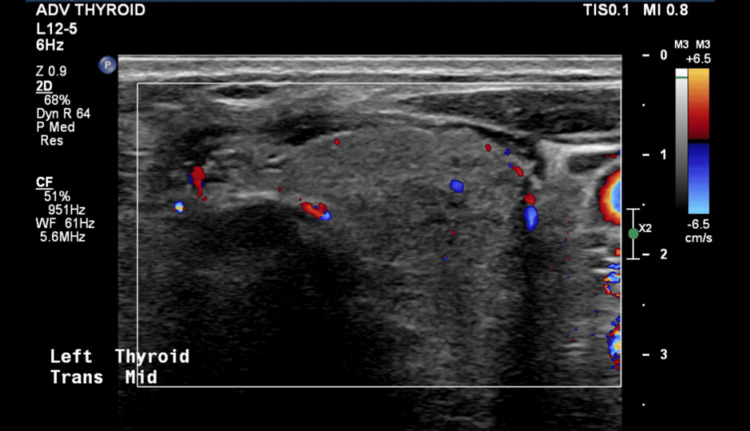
Ultrasound of the left thyroid lobe demonstrating mildly enlarged heterogeneous gland with mildly reduced vascularity
